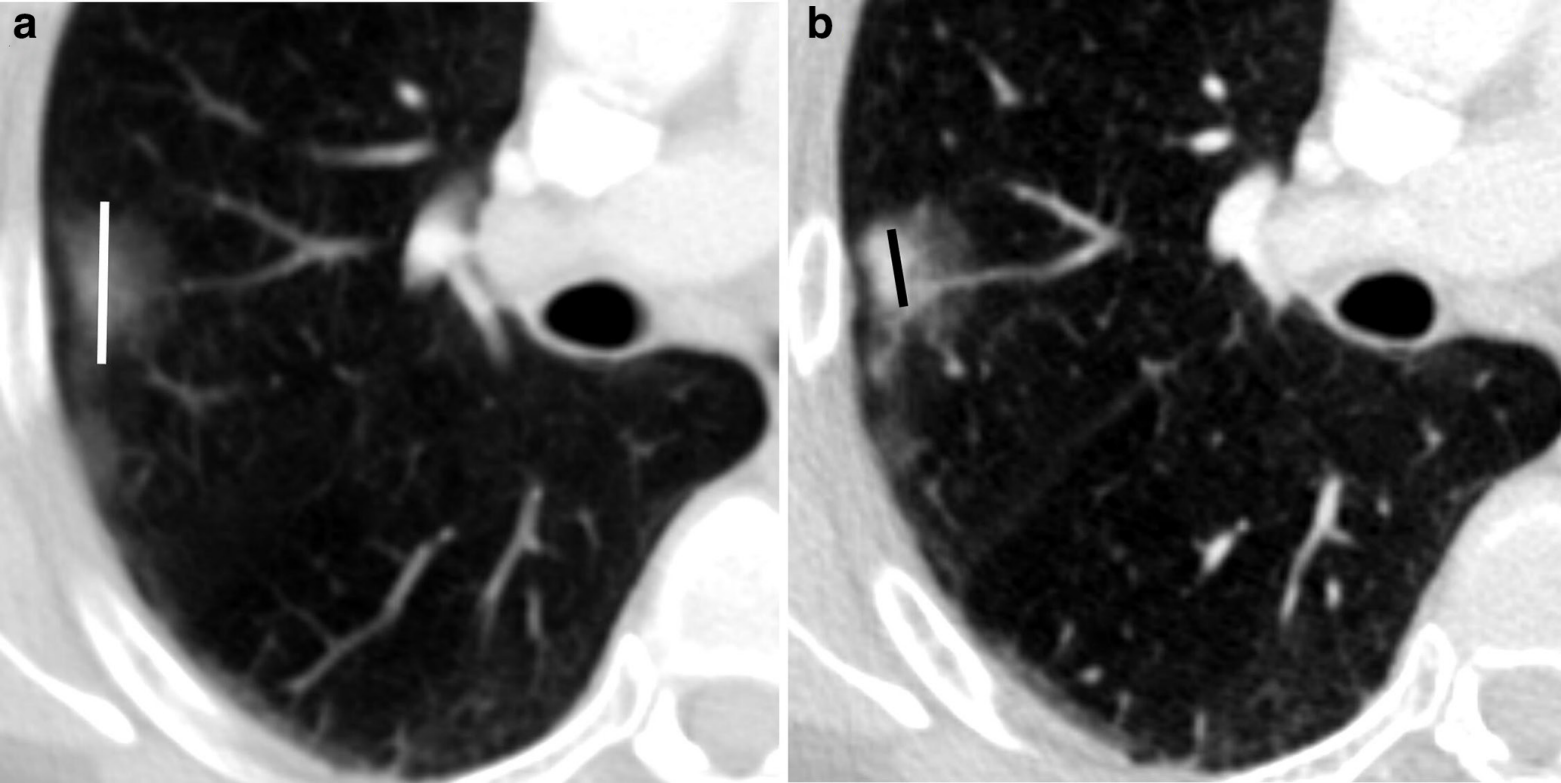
Axial contrast-enhanced CT shows an adenocarcinoma of the right upper lobe. Depending on the slice thickness, the tumour displays pure ground-glass properties on (a) 3 mm reconstructions or solid components on (b) 1 mm reconstructions. It is, therefore, recommended to use only 1 mm reconstructions with sharp reconstruction kernels for lung cancer staging.
